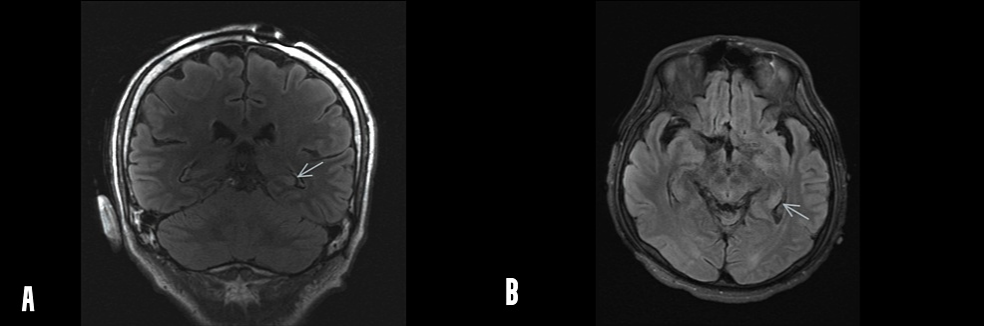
MRI of the brain: FLAIR signal hyperintensity within the dorsal body of the left hippocampus (blue arrow) in the coronal view (A) and axial view (B) MRI - magnetic resonance imaging; FLAIR - fluid-attenuated inversion recovery
